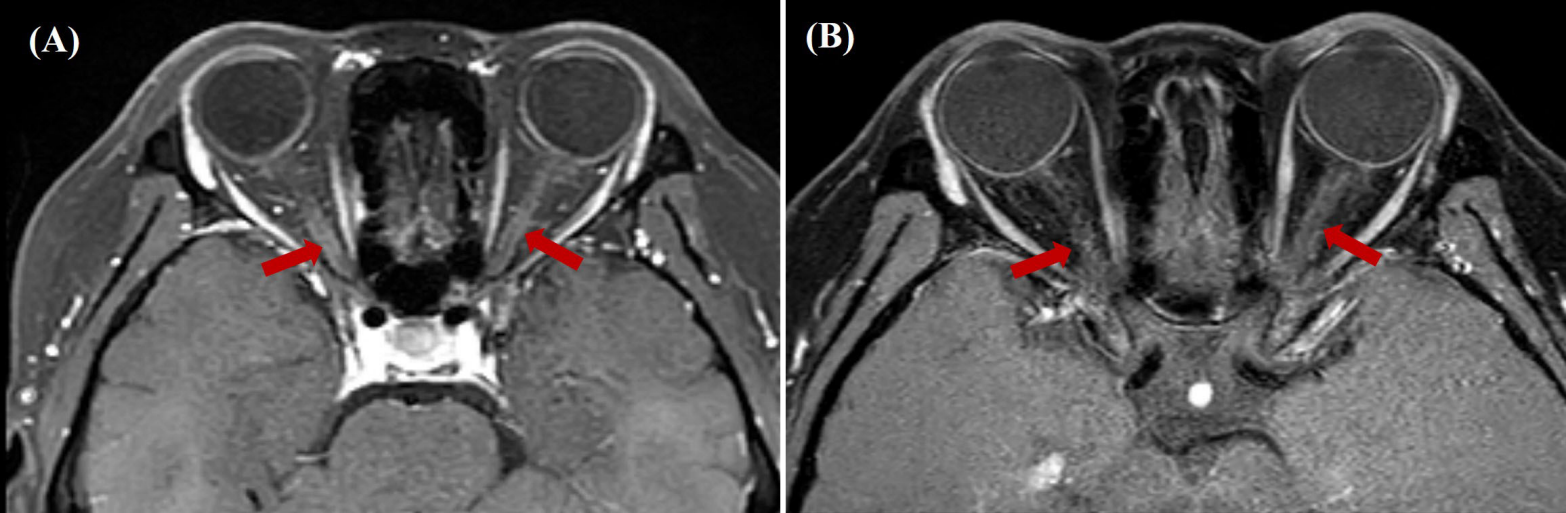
(A) Pre-treatment gadolinium-enhanced orbital MRI (axial STIR sequence) revealed bilateral optic nerve thickening and patchy, heterogeneous enhancement. (B) Post-treatment gadolinium-enhanced orbital MRI (axial STIR sequence) revealed decreased enhancement intensity in the corresponding optic nerve regions compared to baseline. STIR, Short Tau Inversion Recovery.
